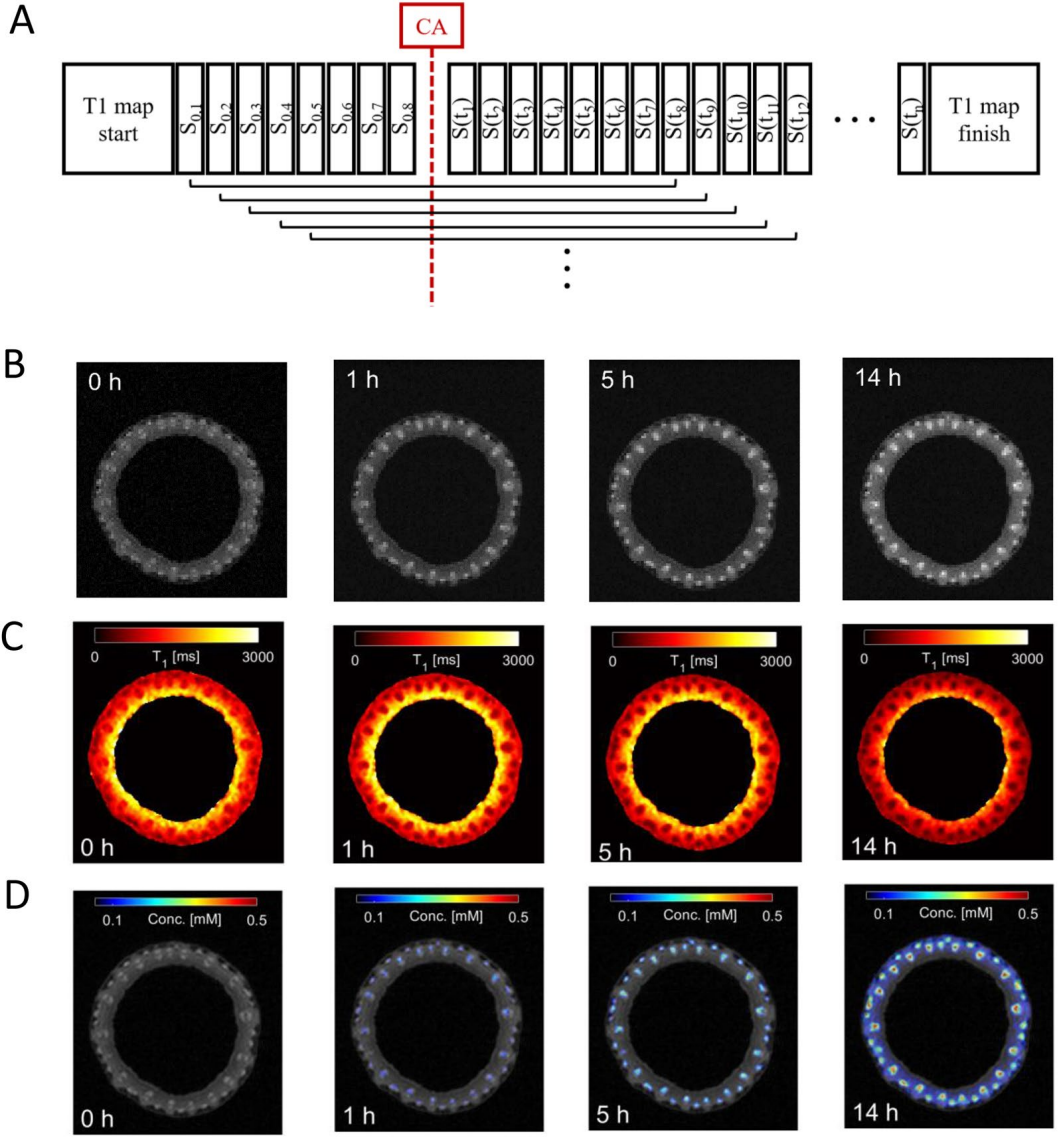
Scheme and application of the pDCE-MRI for monitoring of CA in Barley stem. A Scheme of MRI acquisition for pDCE. B Brightening of the areas corresponding to the vascular bundles due to gadolinium inflow after the CA administration. The time course is exemplified by the T_1_-weighted images of an axial cross section (lowest of the five measured slices) through the sample. C Color-coded images representing the T^1^-maps calculated on the basis of the saturation recovery T^1^-map (shown in Fig. 3A) and the T^1^-weigthed images (shown here in B) by using the pDCE method. A decrease of T^1^ over time can be observed. D Calculated concentration maps of the contrast agent based on the T1-maps from C; background is the 1H-NMR image acquired before CA administration. Accumulation of CA (color-coded) in the areas of the vascular bundles is clearly distinguishable
